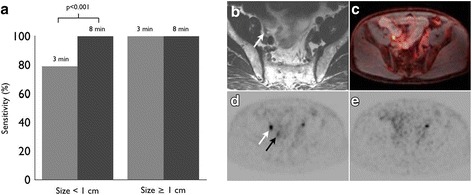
Detection rate for abdominopelvic lymph nodes by size on 3- and 8-min PET acquisitions (a). The 8-min PET is more sensitive for lymph nodes smaller than 1 cm (*p* < 0.001). The 3- and 8-min PET detected all lymph nodes larger than 1 cm. Example, 0.7-cm right external iliac lymph node visible on T2-weighted images (b), fused PET/MR images (c), and on 8-min PET acquisition (d), but not seen on 3-min PET acquisition (e). The right ureter is designated with a black arrow
